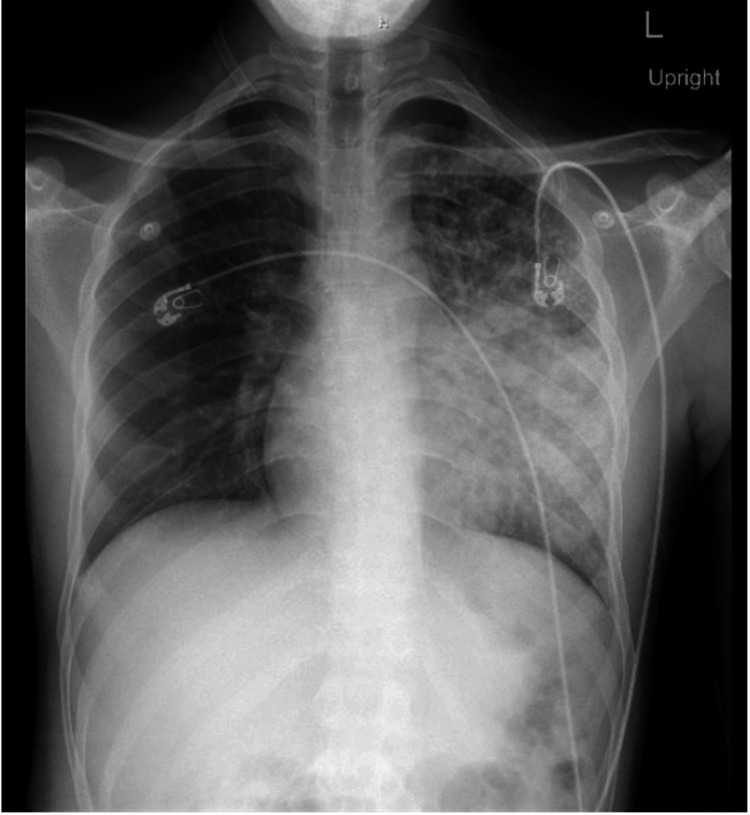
Chest X-ray at initial presentation, revealing diffuse airspace opacities throughout the left lung, as well as left-midlung consolidation.
